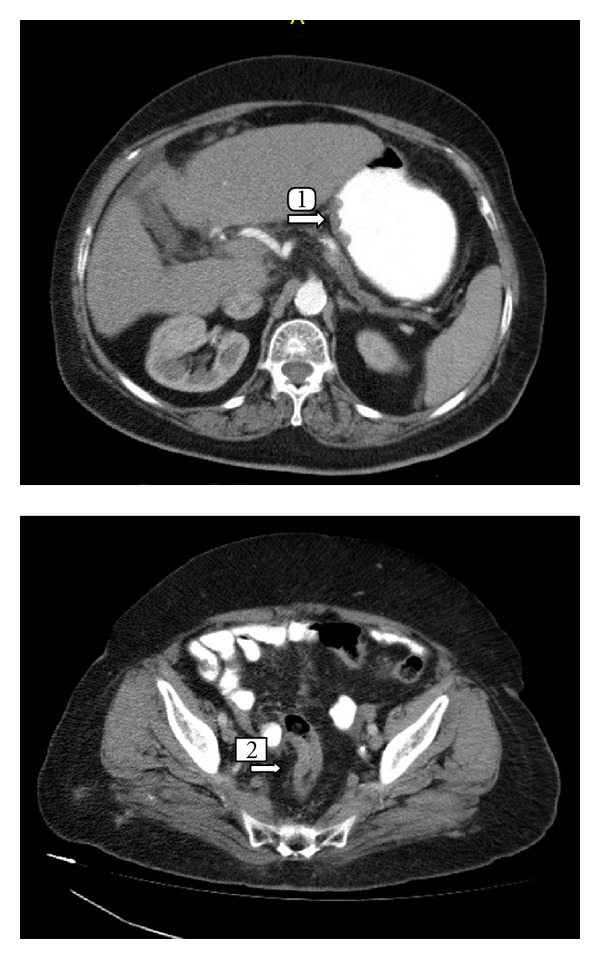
Evaluation of distant metastases by thorax and abdominal CT. No findings were detected in favor of additional metastatic foci in CT scans of thorax or abdomen other than thickening in the gastric wall and asymmetric thickening in the rectal wall. Arrow 1 indicates thickening in the gastric wall and arrow 2 indicates thickening in the rectal wall.
